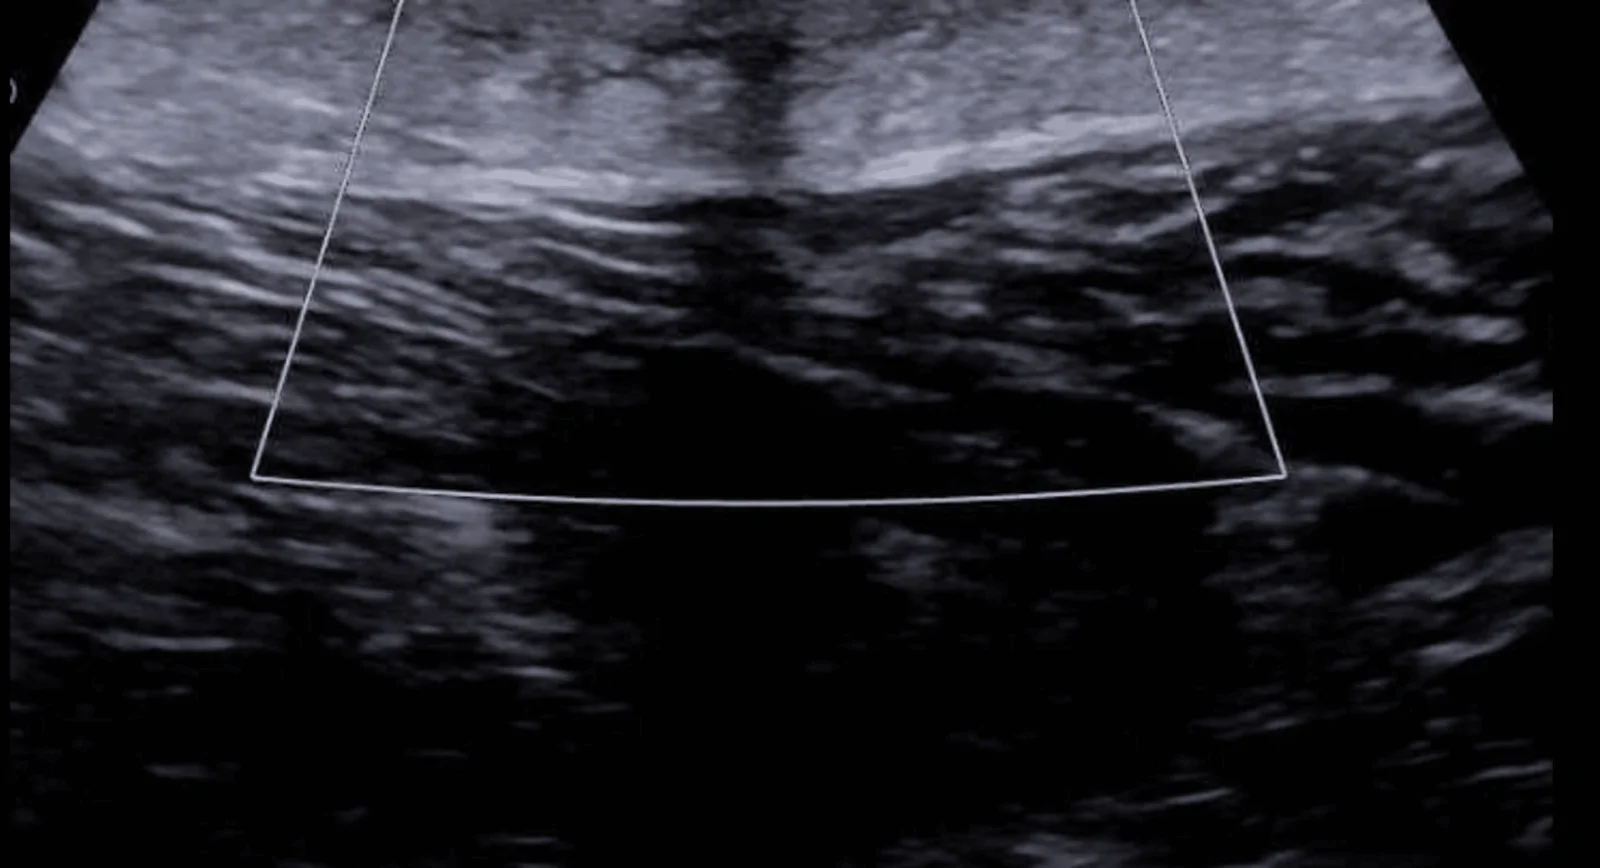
Ultrasonography image showing an area of mild subcutaneous interstitial edema
